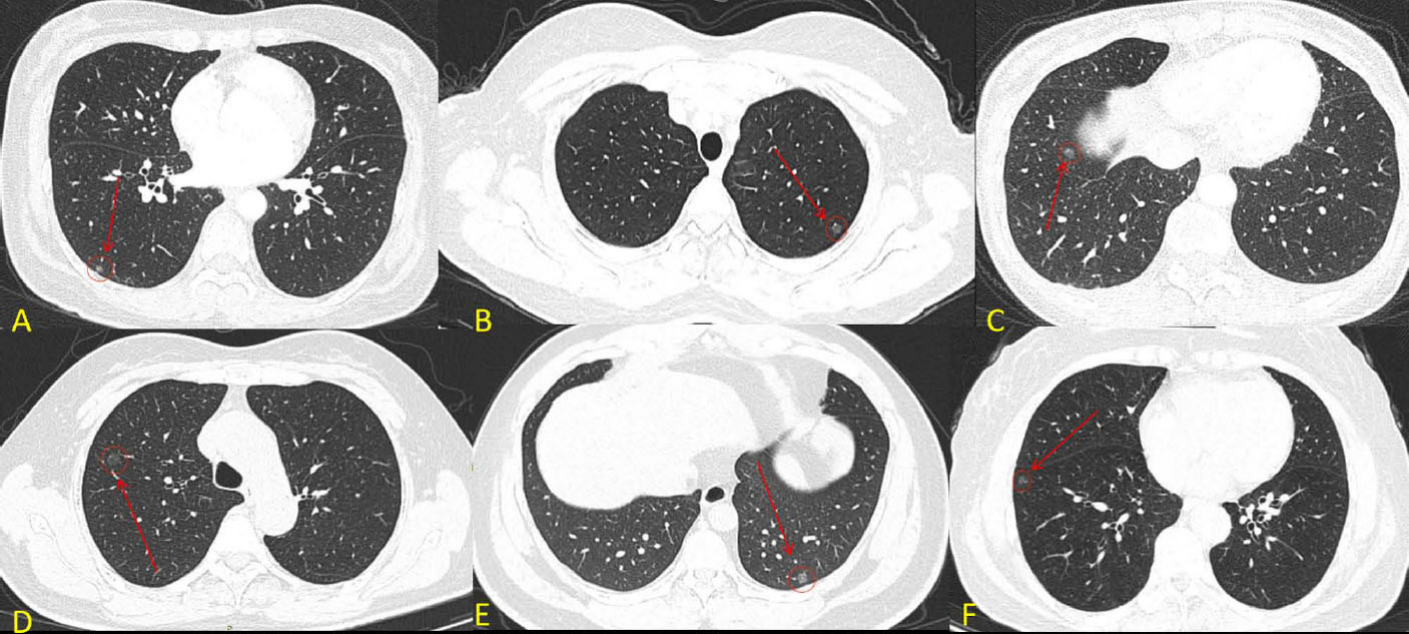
CT images of MPMNs and AIS. A): Female, 48 years old, MPMNs in the right lower lung; B): Female, 53 years old, MPMNs in the left lower lung; C): Female, 48 years old, MPMNs in the right lower lung. D): Female, 61 years old, AIS in the right lower lung; E): Male, 31 years old, AIS in the left lower lung; F): Female, 61 years old, AIS in the right lower lung.
